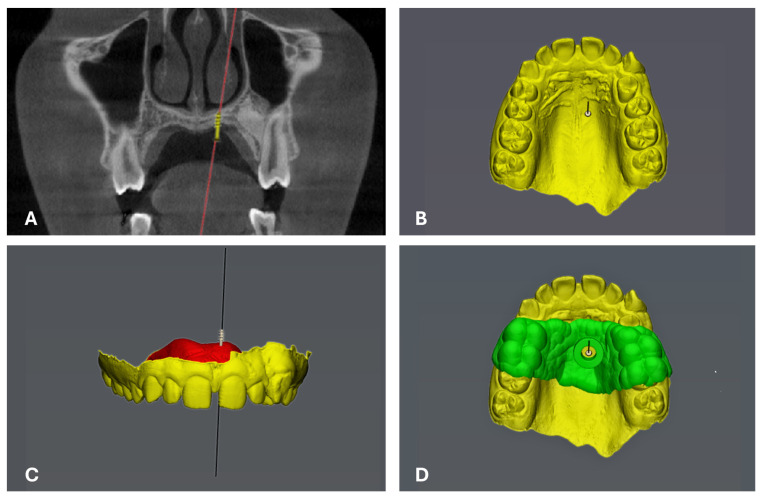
Use of 3D CBCT for palatal TAD insertion: (A) coronal view of final TAD position; (B) occlusal view of the 3D model; (C) frontal view of the 3D model; and (D) surgical guide for TAD insertion.
